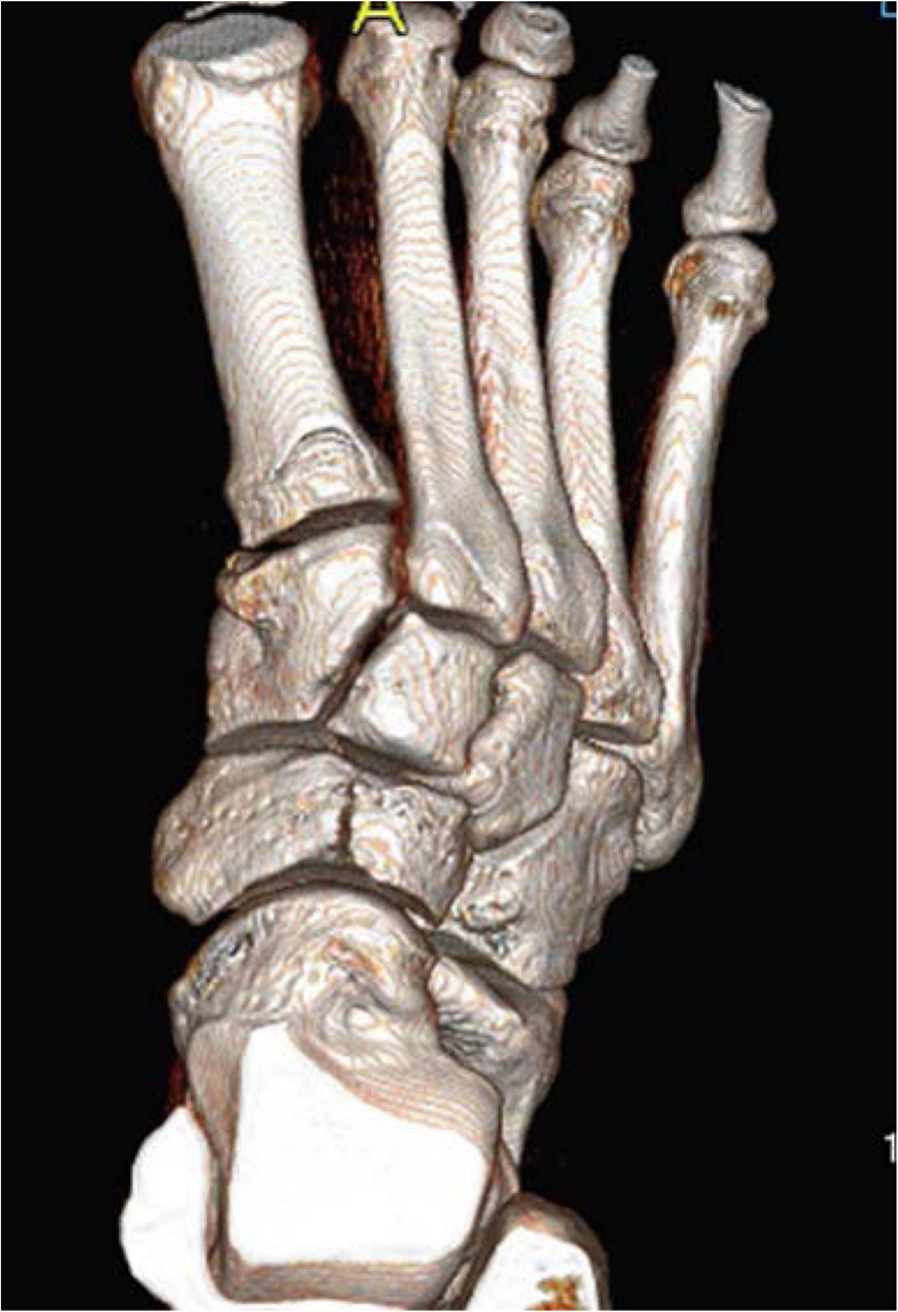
Three-dimensional CT scan of the right foot demonstrating grade III stress fracture of the central one third of the dorsal navicular
